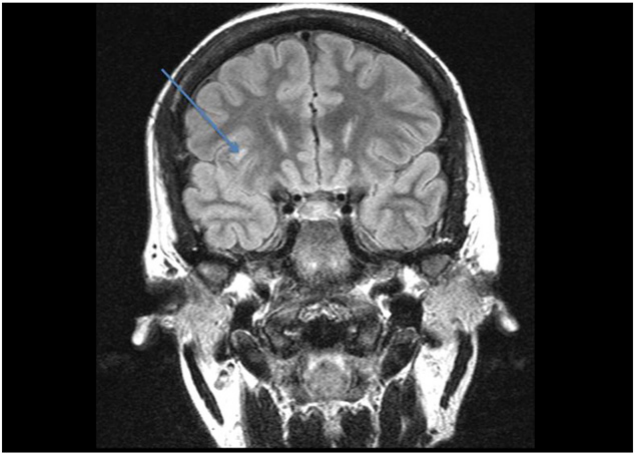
Brain MRI shows small cortical and subcortical lesions at the right inferior frontal lobe.
